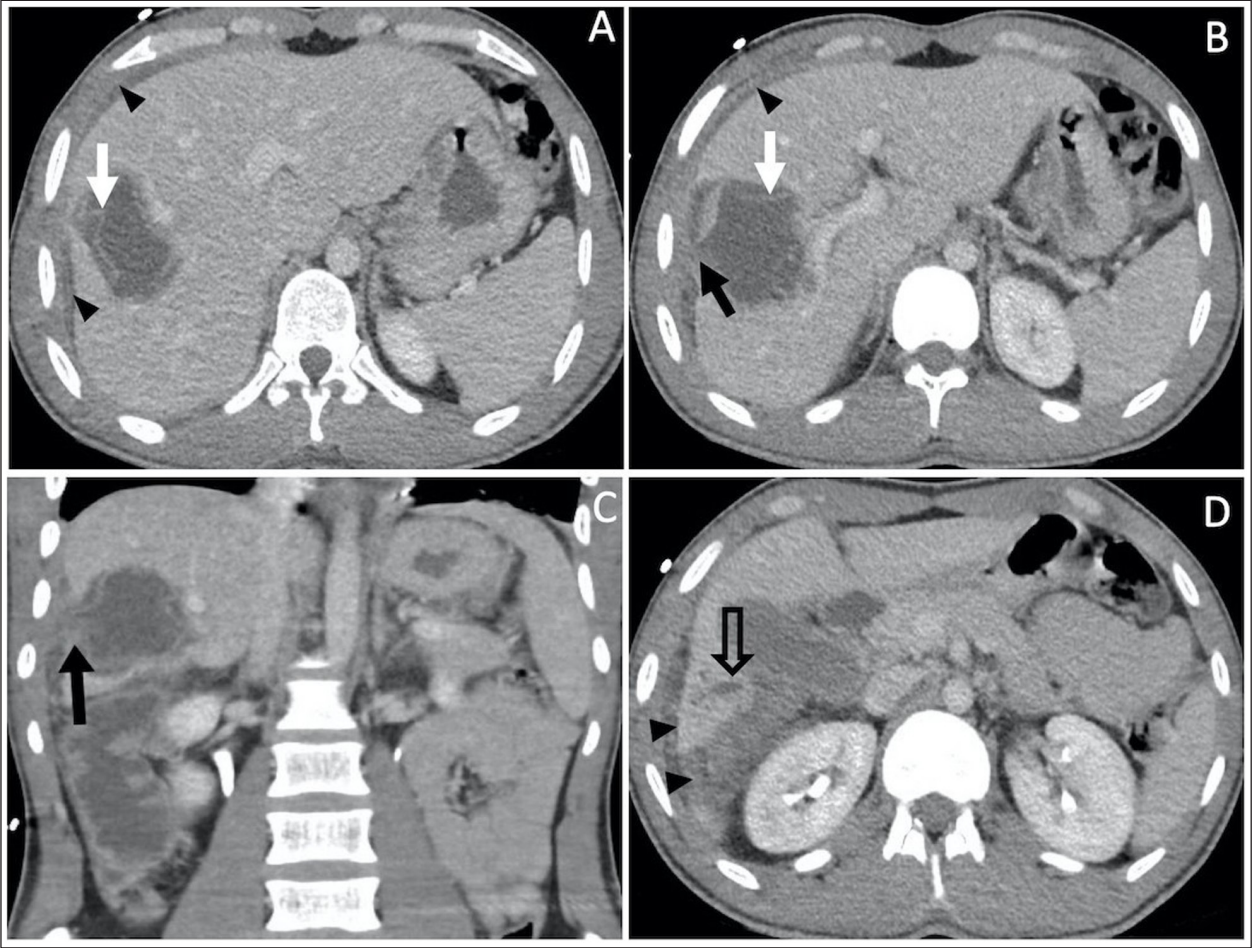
Contrast-enhanced abdominal CT shows a cystic liver lesion with a detached serpentine hyperdense membrane within the cyst, consistent with water lily sign (white arrow in A and B). There is a subcapsular wall defect (black arrow in B and C), fat stranding and free perihepatic fluid (arrowheads in A, B and D). Note the focal intrahepatic bile duct dilatation (hollow arrow in D).
